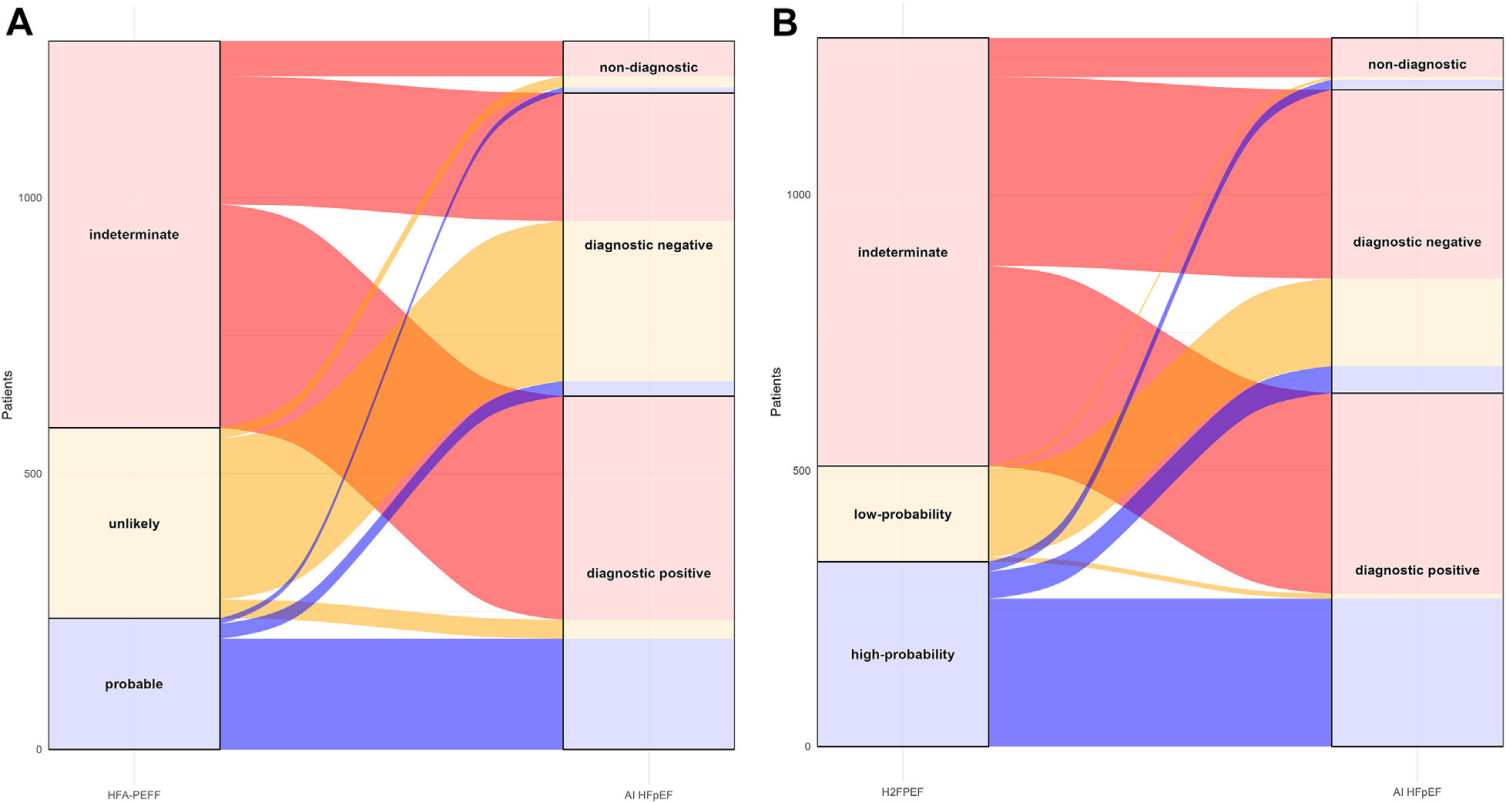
Alluvial Plot Demonstrating Reclassification of Patients Using Clinical Scores Compared to the AI HFpEF Model Patients in the independent testing data set were given a categorical score based on relevant functional and morphological echocardiographic, and biomarker parameters. Patients were categorized as unlikely (0 or 1), indeterminate (2-4), or probable (5-6) likelihood of heart failure with preserved ejection fraction for the Heart Failure Association-Pretest Assessment, Echocardiographic and Natriuretic Peptide Score, Functional Testing, and Final Etiology (HFA-PEFF) score (A), and low-probability (0 or 1), indeterminate (2-5), or high-probability (6-9) of heart failure with preserved ejection fraction for the Heavy, Hypertensive, Atrial Fibrillation, Pulmonary Hypertension, Elder, and Filling Pressure (H2FPEF) score (B). AI = artificial intelligence; HFpEF = heart failure with preserved ejection fraction.
